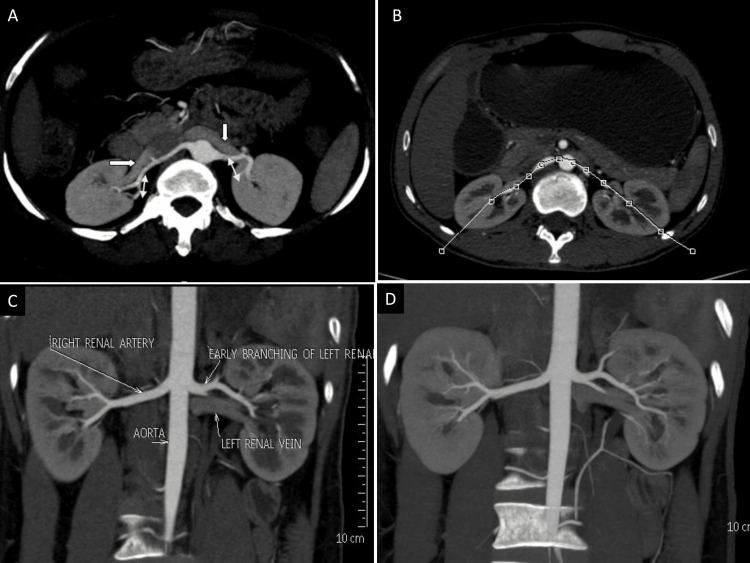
Computed tomography angiographic evaluation of renal arterial anatomy (A) Post-processing axial maximum intensity projection image showing bilateral renal veins (→) lying anterior to renal arteries (⇔)(B) Axial computed tomography image displaying curved lined drawn at the level of renal artery to create a curved plane (c) Curved coronal reformatted image showing bilateral single renal artery with early branching of left renal artery (D) Curved maximum intensity projection image displaying renal arteries branches better.
